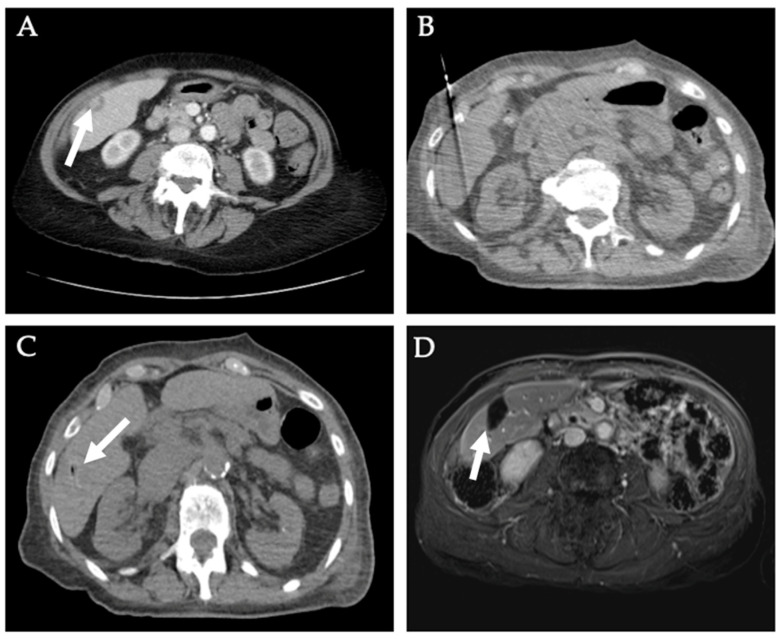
Microwave ablation of hepatic metastatic leiomyosarcoma. A 73-year-old female patient with a 2.1 × 1.5 cm sub-capsular metastatic leiomyosarcoma (white arrow) in segment V (A) on CT scan. The patient underwent chemotherapy followed by MWA. (B) Probe placement and (C) immediate post-ablation image of the right hepatic lobe lesion. The patient then underwent proton therapy of inferior vena cava mass and left hepatic metastasis. (D) Six-month follow-up MRI shows complete response.
